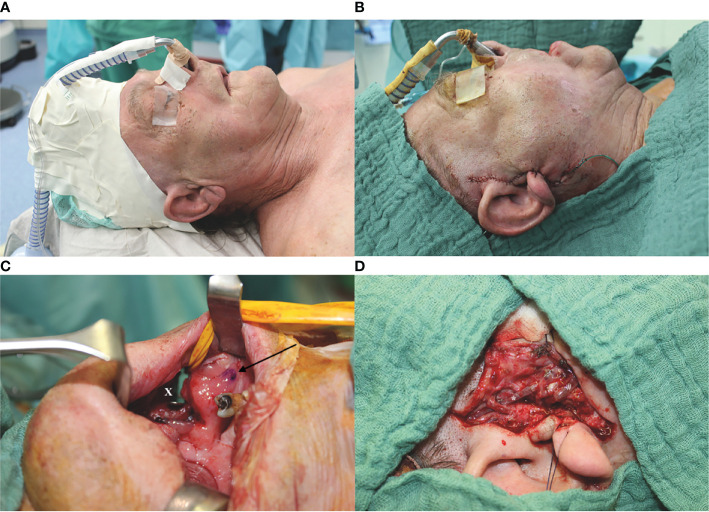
Exemplary case demonstration with temporally anastomosed one-segmented osteomyocutaneous fibula free flap because of osteoradionecrosis of the right mandibular body. Preoperative CT angiography revealed no reasonable cervical external carotid branches for microvascular anastomosis due to previous operations: (A) preoperative situation; (B) postoperative situation without cervical scars after mandibular reconstruction; (C) preparation of the transfacial subcutaneous tunnel (x) with careful preservation of the parotid duct, its intraoral ostium (arrow), and the accompanying buccal branch of the facial nerve; and (D) temporal anastomoses with the superficial temporal artery and vein (STA/V). The STV has been divided to allow the anastomoses of two veins, one draining retrograde to the superficial scalp (*) and the other orthograde into the deep cervical venous system (#).
